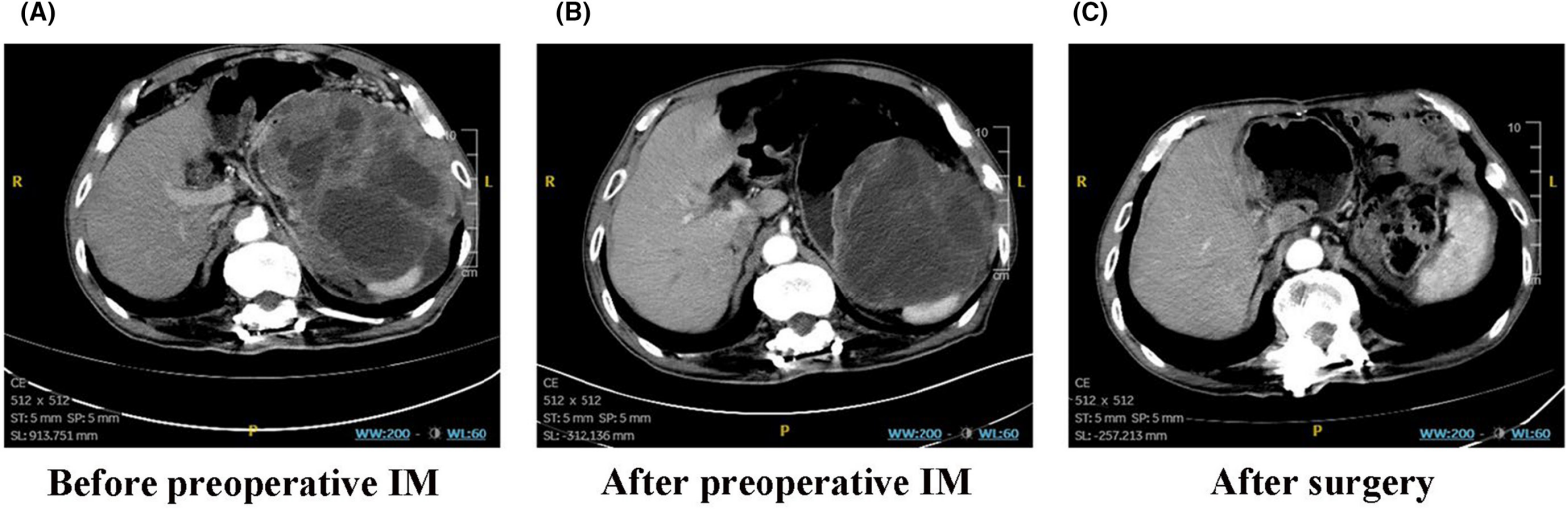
Contrast‐enhanced abdominal CT imaging in a 73‐year‐old male patient with locally advanced gastric gastrointestinal stromal tumors who received 8 months of preoperative imatinib. (A) Before preoperative imatinib treatment (maximum tumor diameter: 19 cm); (B) After 8 months of preoperative imatinib treatment (maximum tumor diameter: 14 cm); (C) 3 months after local gastrectomy.
